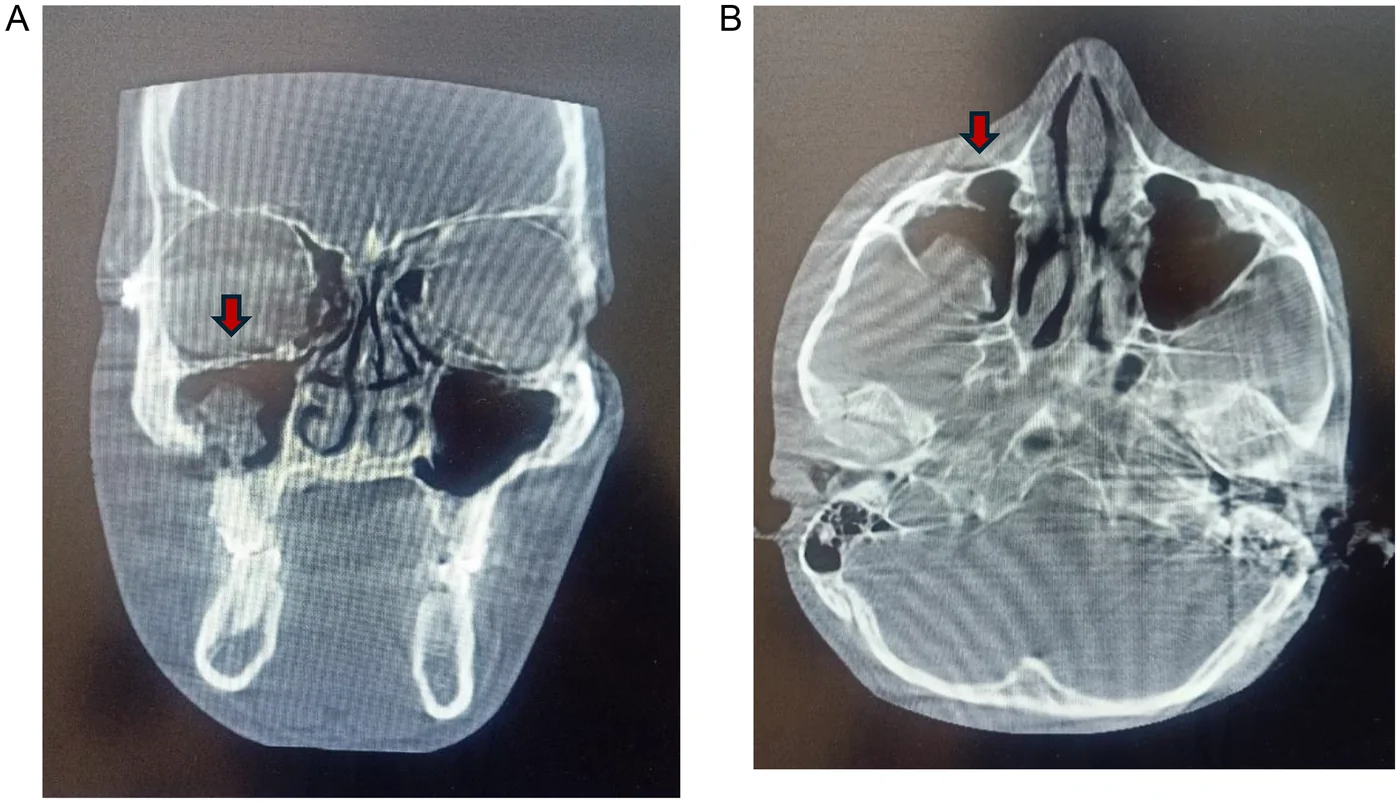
(A) 2 months postoperative CT scan (coronal view). (B) 2 months postoperative CT scan (axial view).
